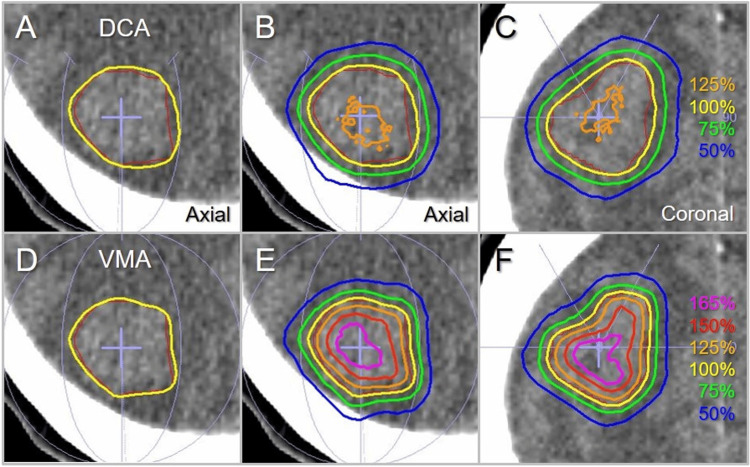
Comparison of the dose distributions for GTV of 9.54 cc. The images show computed tomographic images (axial views: A, B, D, E; coronal views: C, F) of the case harboring a brain metastasis in the right parietal lobe (A-F), onto which the GTV outline in red, arc arrangements in light purple, and the representative isodose lines for DCA (A-C) and VMA (D-F) are superimposed. The isodose lines are shown as the relative values to the prescription dose (100%) in yellow. GTV: gross tumor volume; DCA: dynamic conformal arcs; VMA: volumetric modulated arcs.
